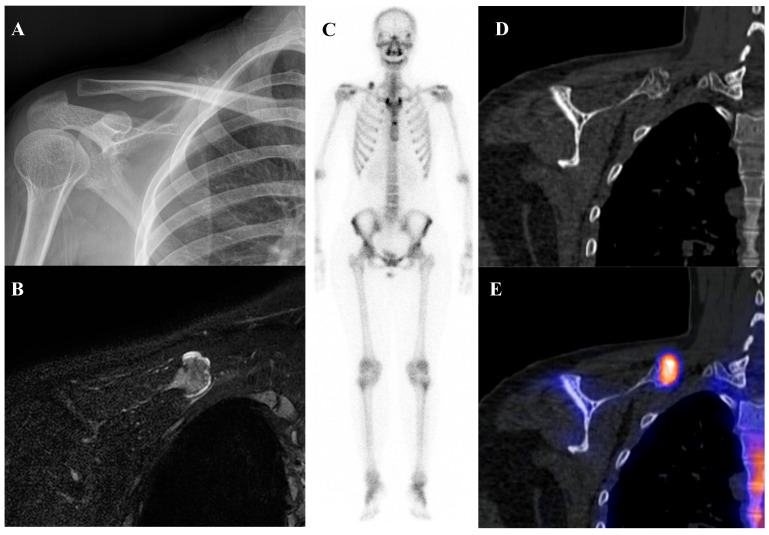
A 16-year-old female with osteochondroma of the right scapula. (A) Plain radiograph shows a protruding mixed sclerotic mass at the scapular cap. (B) T2-weighted fat-suppressed magnetic resonance imaging demonstrates a pedunculated, lobulated osseous lesion arising from the scapular cap, with continuity of the cortex and medulla and a thin, T2-bright enhancing cartilaginous cap. (C) Bone scintigraphy shows focal uptake at the lesion site. (D,E) Bone single-photon emission tomography/computed tomography demonstrates focal tracer uptake at the bone–cartilage junction.
